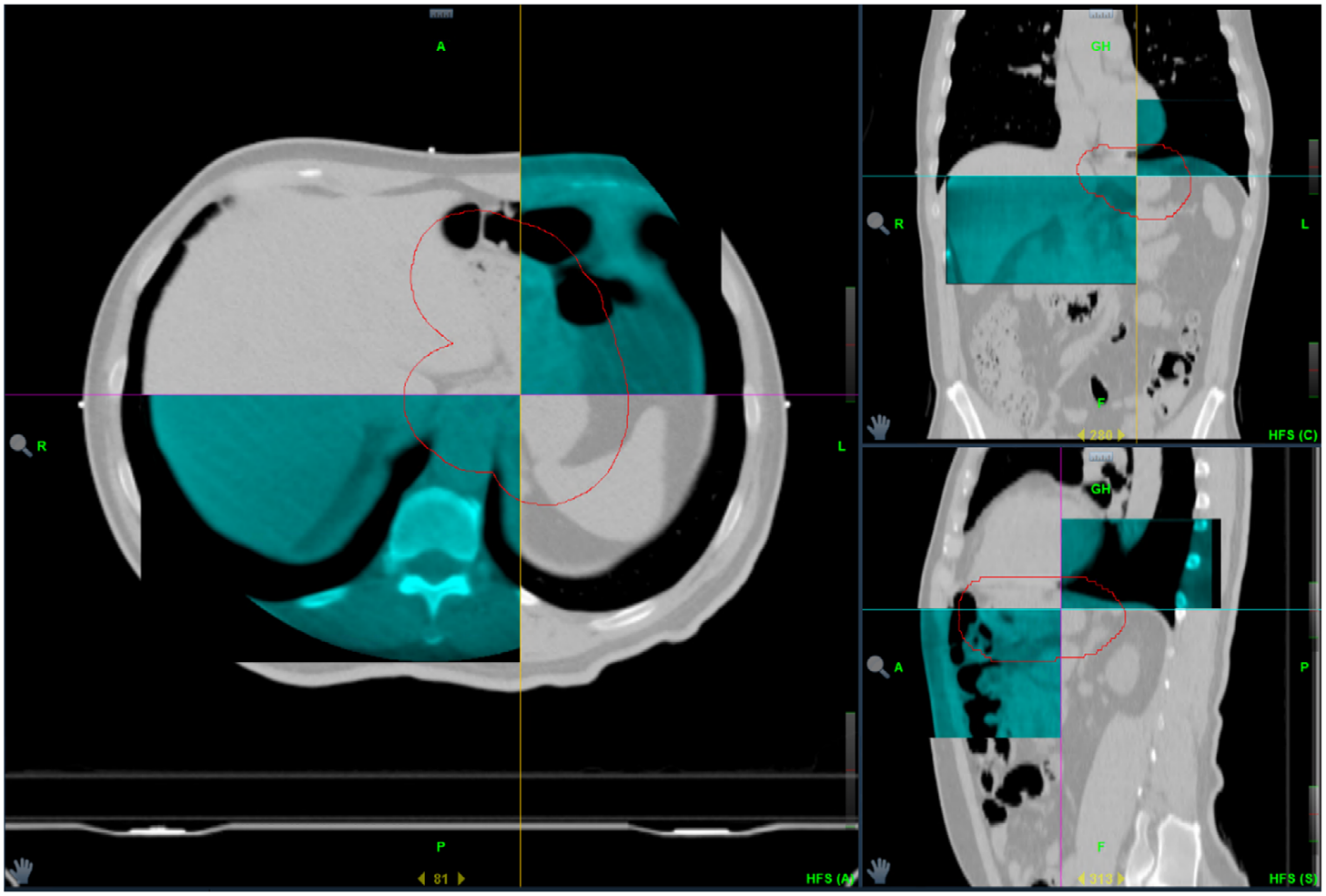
Axial, sagittal and coronal planes of a set of typical matching images for positional verification. grey color: planning CT images; cyan colour: kVCT images taken during image guidance.
